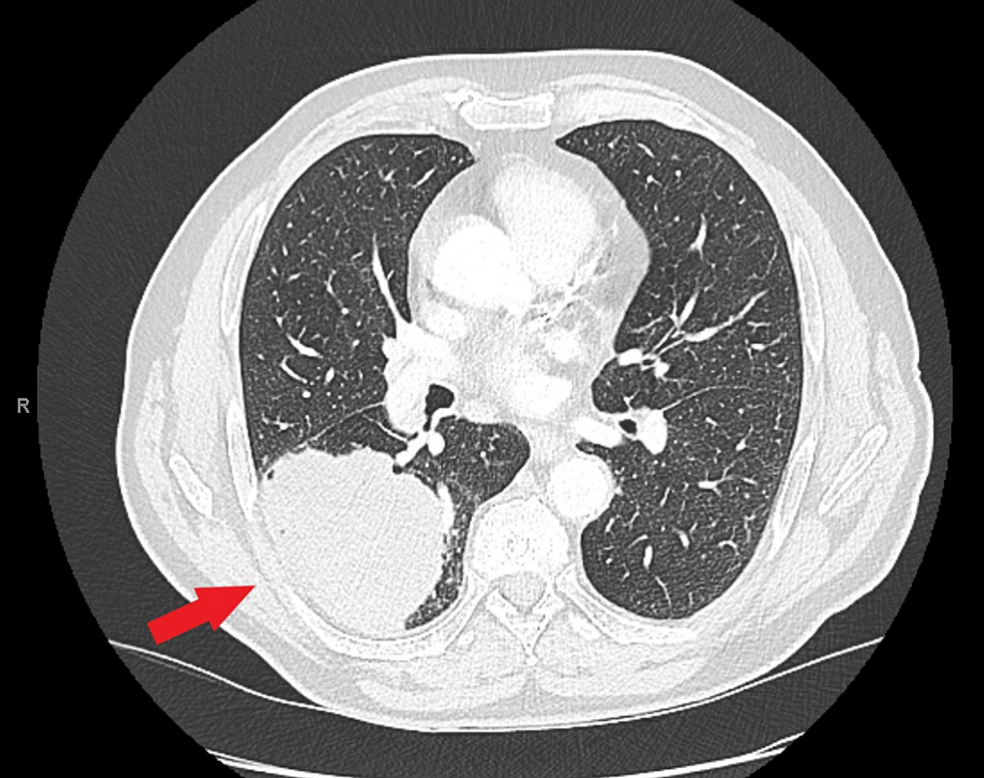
Chest CT scan Chest CT demonstrating a 9-centimeter pulmonary mass located in the right lower lobe (red arrow)
